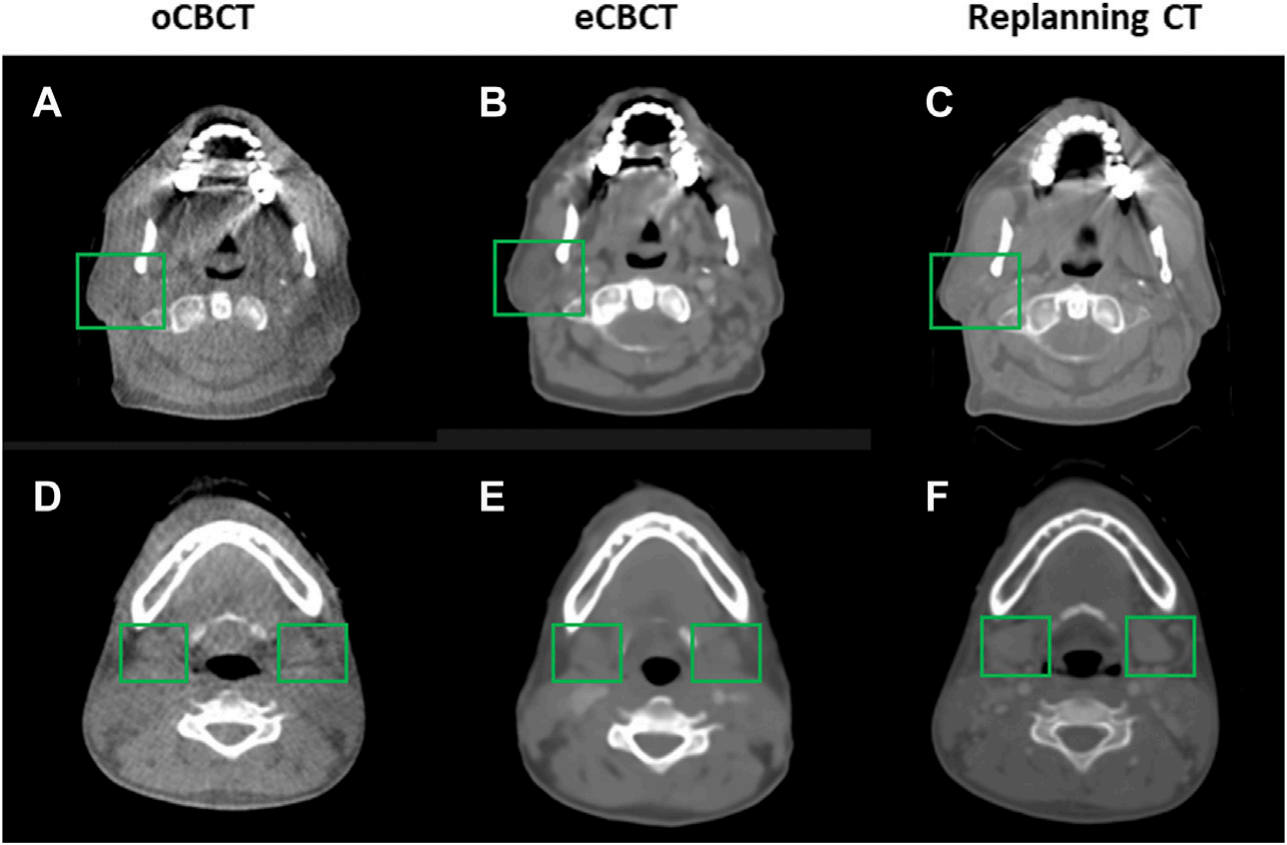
Comparison of image quality for one representative patient. eCBCT has lower image noise and less streak artifacts in the soft tissue region than the oCBCT. eCBCT also has higher image contrast than oCBCT for parotid and submandibular gland areas (see green box).
